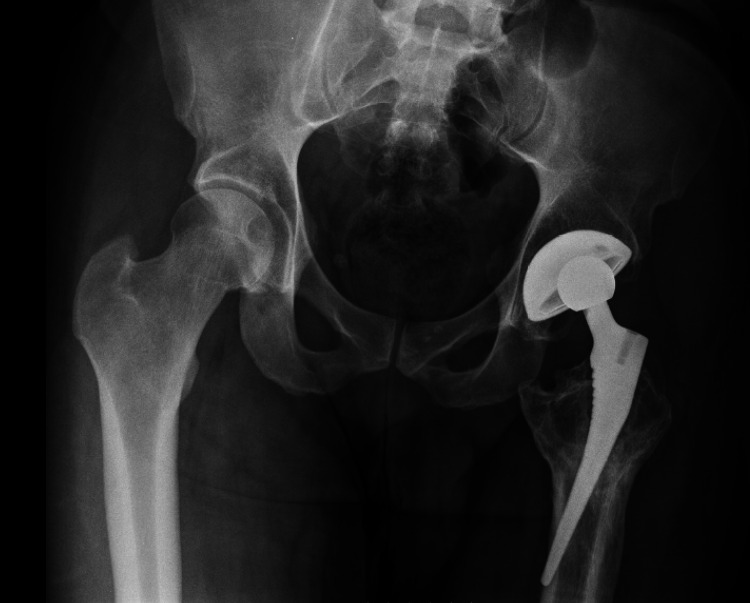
Anteroposterior pelvic radiographic image demonstrating pelvic tilt
